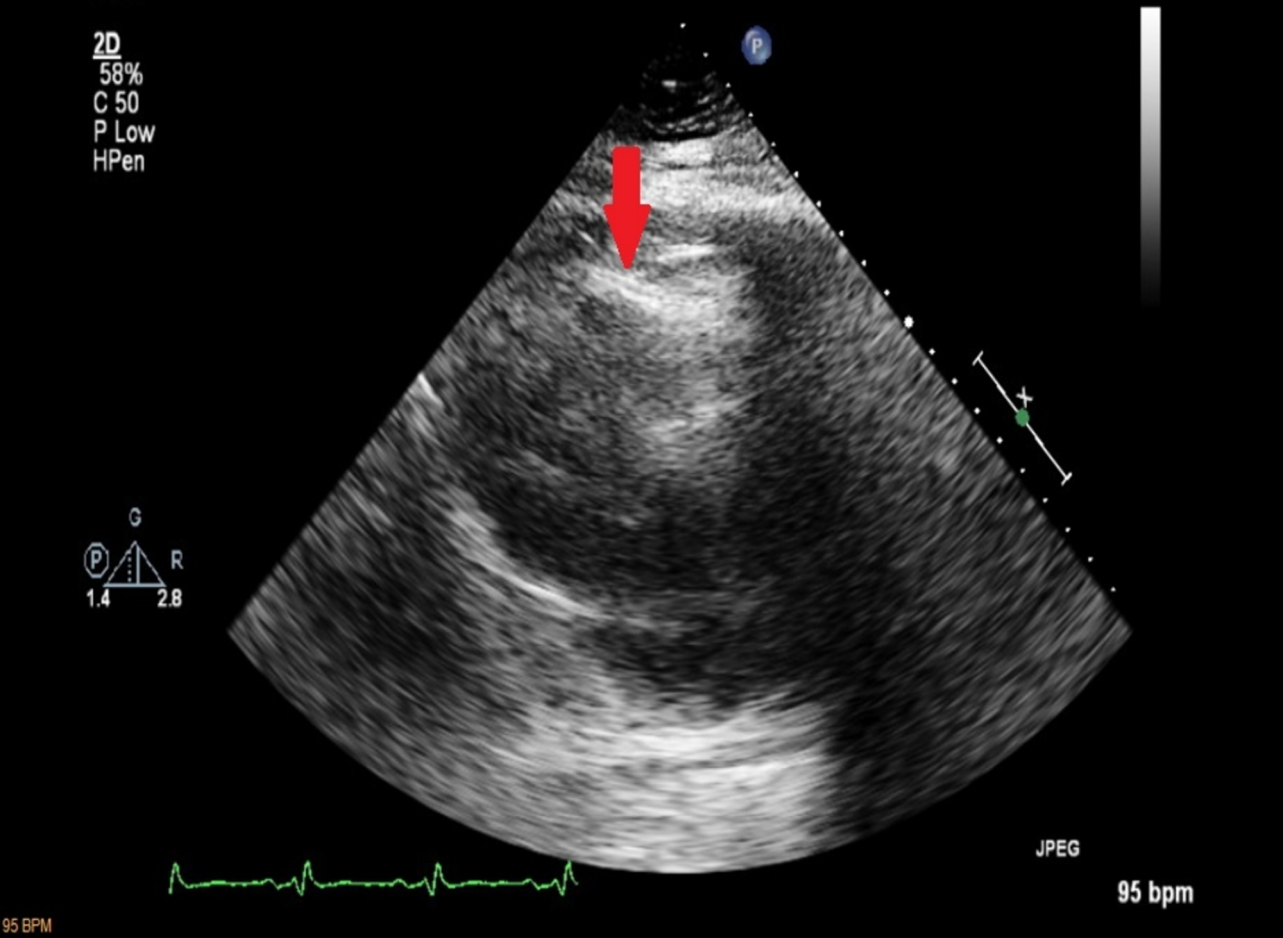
Echocardiogram Apical four chamber echocardiogram view showing mild right ventricular dilatation (red arrow).
